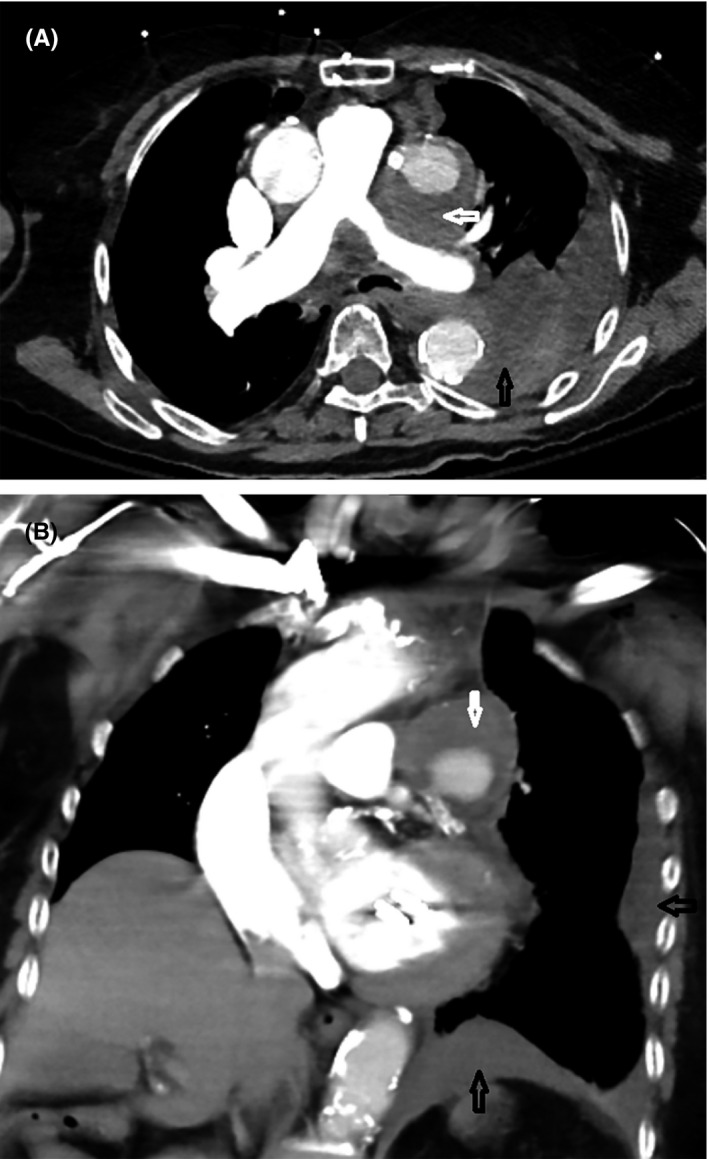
A, Contrast CT angiogram of chest (transverse) showing a 45 × 40 mm pseudoaneurysm of SVG to OM with large clot burden (white arrow) along with large left‐sided hemothorax (black arrow). B, Contrast CT angiogram of chest (coronal) showing a 45 × 40 mm pseudoaneurysm of SVG to OM with large clot burden (white arrow) along with large left‐sided hemothorax (black arrow)
